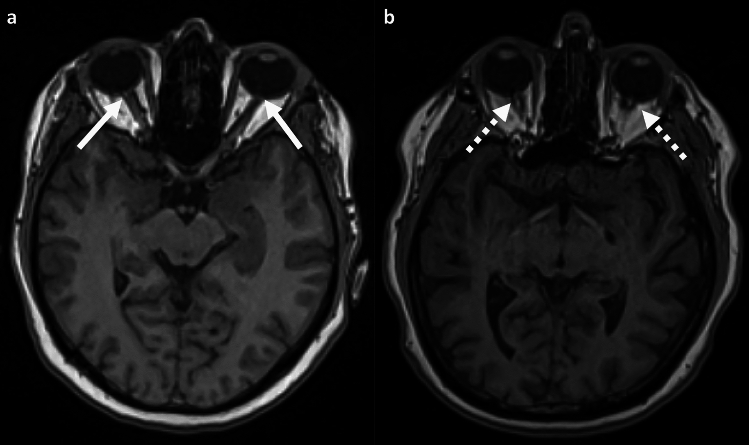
Variations in the shape of the optic disc on axial T1-weighted images. (a) 42-year-old male participant with flat optic disc (white arrow) and straight course of the optic nerve. (b) 43-year-old female participant with bulging of the optic disc (dotted white arrow), enlarged subarachnoid space and meandering course of the optic nerve.
